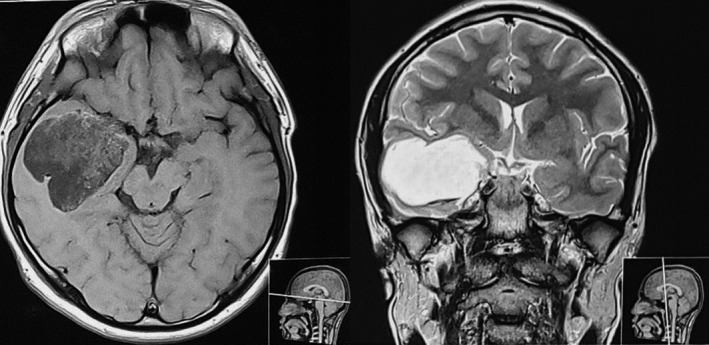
T1‐MRI (Axial section) showing intraaxial mass of variable intensity in the right temporal pole compressing adjacent ambient and Sylvian cistern (Left) and T2‐MRI (Coronal section) with bright signal intensity in the right temporal region (Right)
